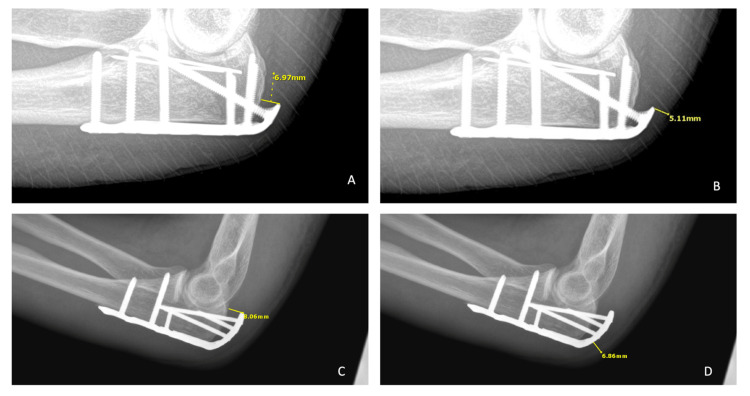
Sample of standardized radiographic measurements Synthes plate prominence (A) and soft tissue thickness (B); Acumed plate prominence (C) and soft tissue thickness (D)
